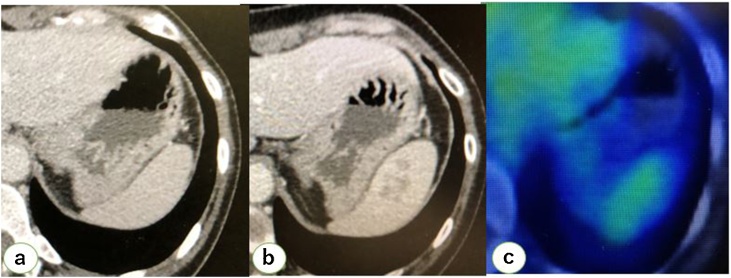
CT and FDG-PET findings. (a–b) CT showing a relatively well-circumscribed, heterogeneously enhanced round mass measuring 25 mm [a) 3 years previously and b) before the present surgery]. (c) FDG-PET showing a slight uptake in the splenic lesion; no other significant findings indicated recurrence at other sites.
